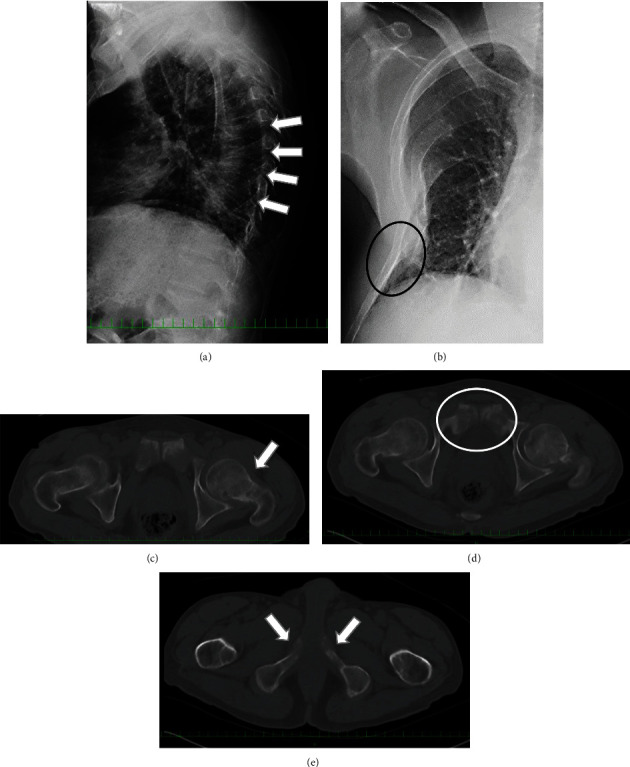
Radiographs taken in 2007 showing multiple vertebral fractures (a, white arrows) and right rib malunion (b, black circle). A left femoral neck insufficiency fracture (c, white arrow) and deformity of both pubes (d, white circle) and ischia (e, white arrows) are shown in computed tomography images in 2007.
